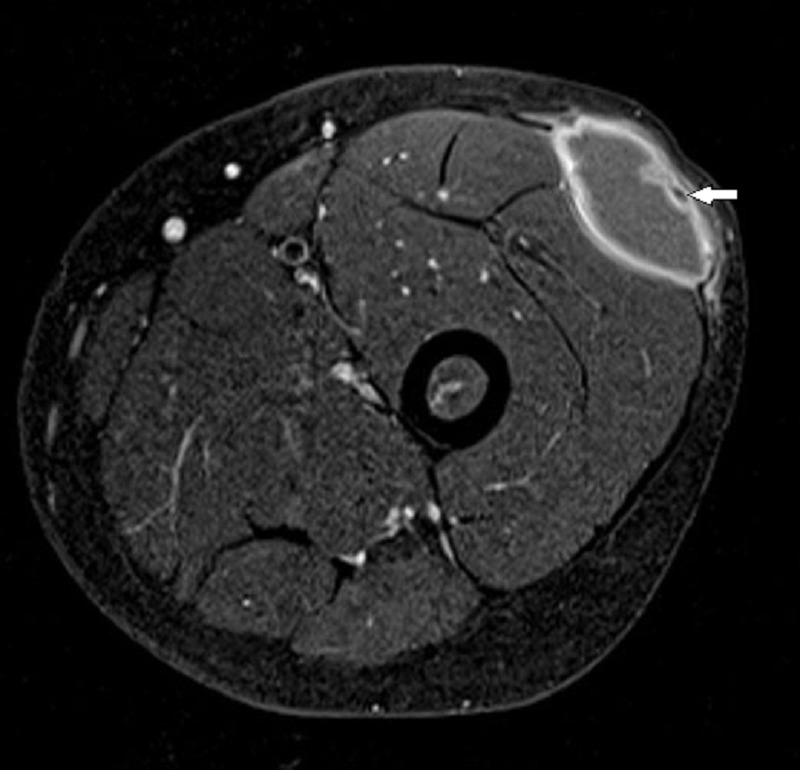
Figure 1: MRI showing lesion with post-contrast wall enhancement. Arrow showed scolex.
